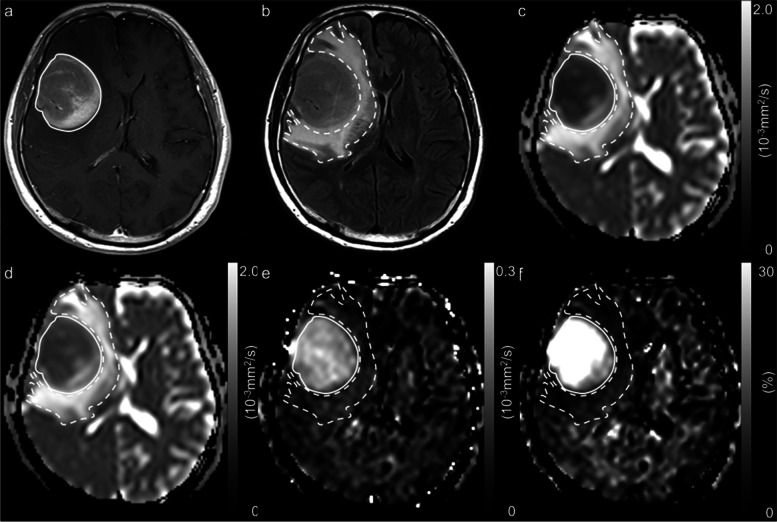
A 67-year-old man with primary central nervous system lymphoma. A contrast-enhanced T1-weighted image with a ROI (solid line) (a), a fluid attenuated inversion recovery image with a ROI (dotted line) (b), an ADC map derived from pulsed gradient spin-echo (PGSE) DWI at an effective diffusion time (Δ_eff_) of 44.5 ms (c), an ADC map derived from oscillating gradient spin-echo (OGSE) DWI at an Δ_eff_ of 7.1 ms (d), and maps of the ADC change between PGSE DWI and OGSE DWI (cADC) (e) and the relative ADC change between PGSE DWI and OGSE DWI (rcADC) (f). The ADC values in the tumor appear higher at short Δ_eff_ values than at long Δ_eff_ setting. Large changes in the cADC and rcADC are noted between the OGSE and PGSE sequences in the tumor
